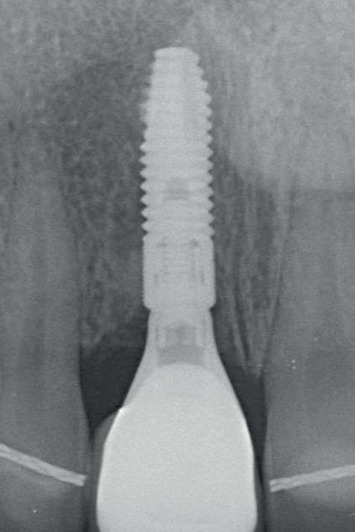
Radiographic view of the implant and definitive restoration at the final follow-up appointment.
